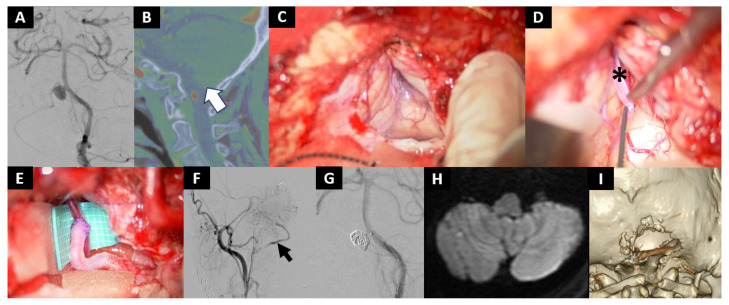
Case 8: A right BA–AICA aneurysm treated by a hybrid strategy combining bypass reconstruction and coil embolization. (A) Digital subtraction left vertebral angiogram shows right BA–AICA aneurysm with an AICA–PICA variant originating from the neck of the aneurysm. (B) Sagittal section of the CT angiogram shows that the distal segment of the AICA-PICA courses caudal to the tonsil (white arrow). (C–G) Intraoperative photographs obtained during the combined bypass reconstruction and coil embolization in the hybrid operating room. (C) A limited craniotomy is made below and medial to the inferior nuchal line. The mastoid air cell is not opened. (D,E) The bypass was performed at the tonsillar segment of the AICA-PICA (*) without retraction of the cerebellum. (F) Intraoperative digital subtraction left vertebral angiogram confirms good bypass patency (black arrow). (G) Subsequently, the aneurysm and the origin of AICA are embolized with coils. (H) Postoperative MRI diffusion-weighted imaging shows no ischemic infarction. (I) 3D-reconstructed plain CT confirms that a small craniotomy is performed as planned and the mastoid air cell is not opened.
